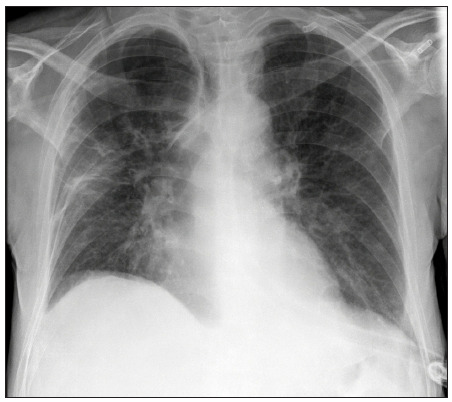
Lung radiograph of the patient in the first report, indicating pneumonia caused by *Bordetella bronchiseptica*.
